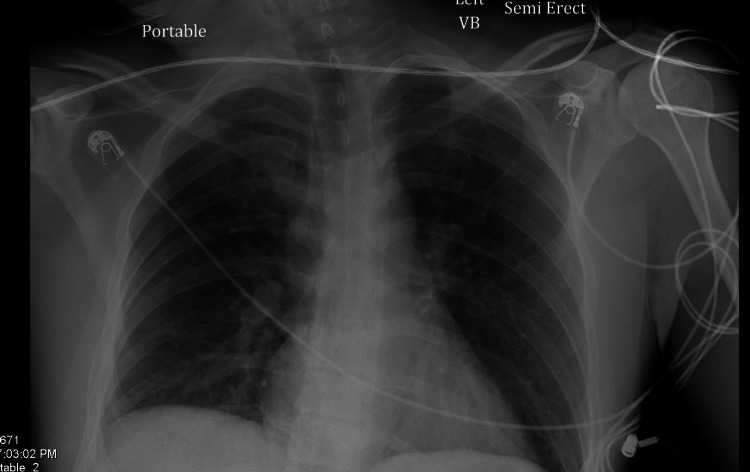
Portable chest X-ray significant for patchy increased density in the right base
